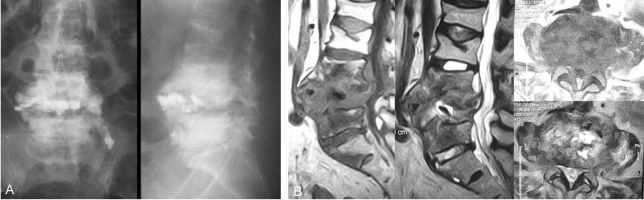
At 34 months after the vertebroplasty (the first visit to our hospital), a roentgenographic image (A) and MRI (B) revealed a sclerotic change of the vertebral body, with anterior displacement of the bone cement, and multiple paraspinal and intraspinal abscesses with invasion of the spinal canal.
